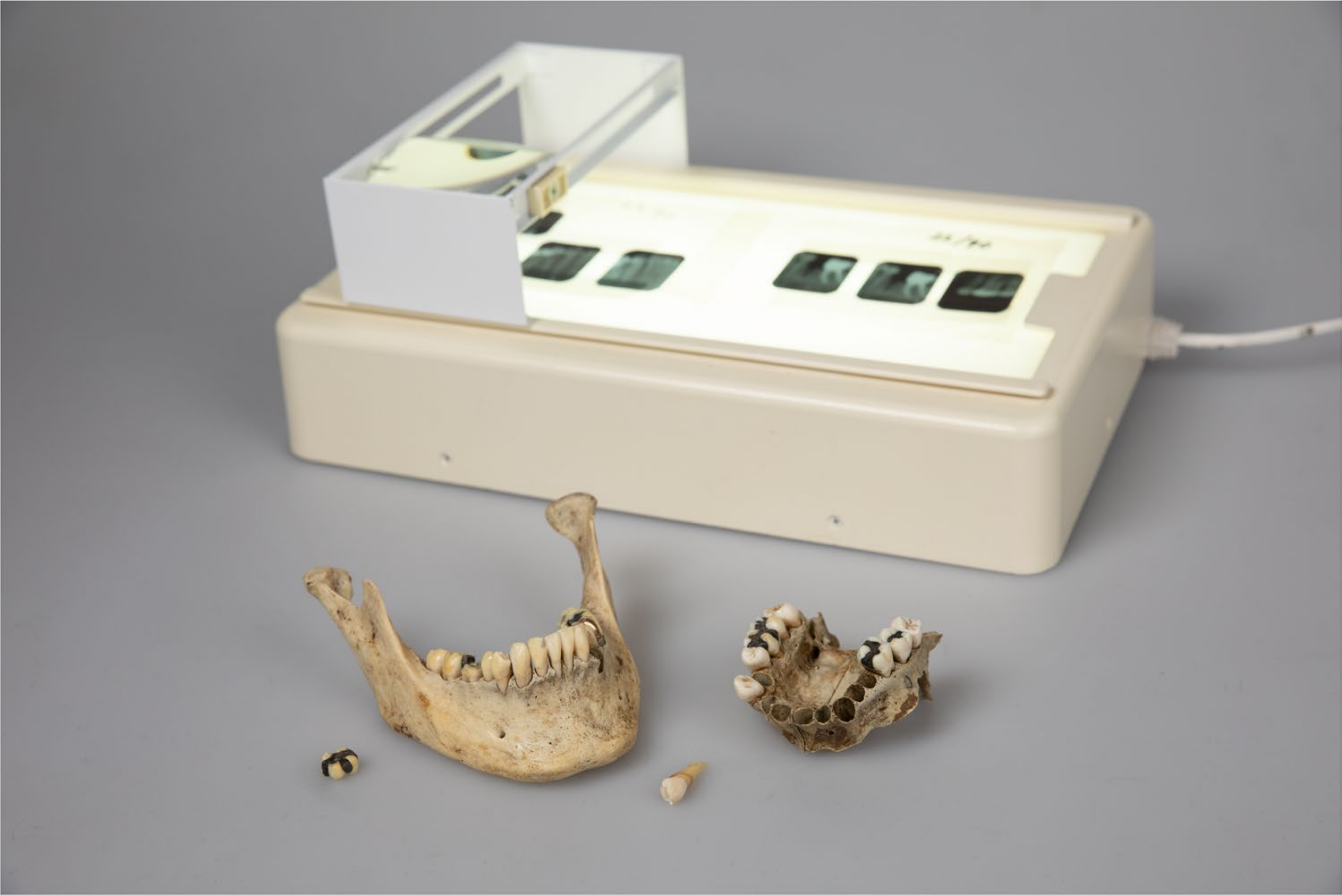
The educational material used prior to our new digitalised educational programme; lightbox with analogue intraoral radiographs and deteriorated material from human remains
